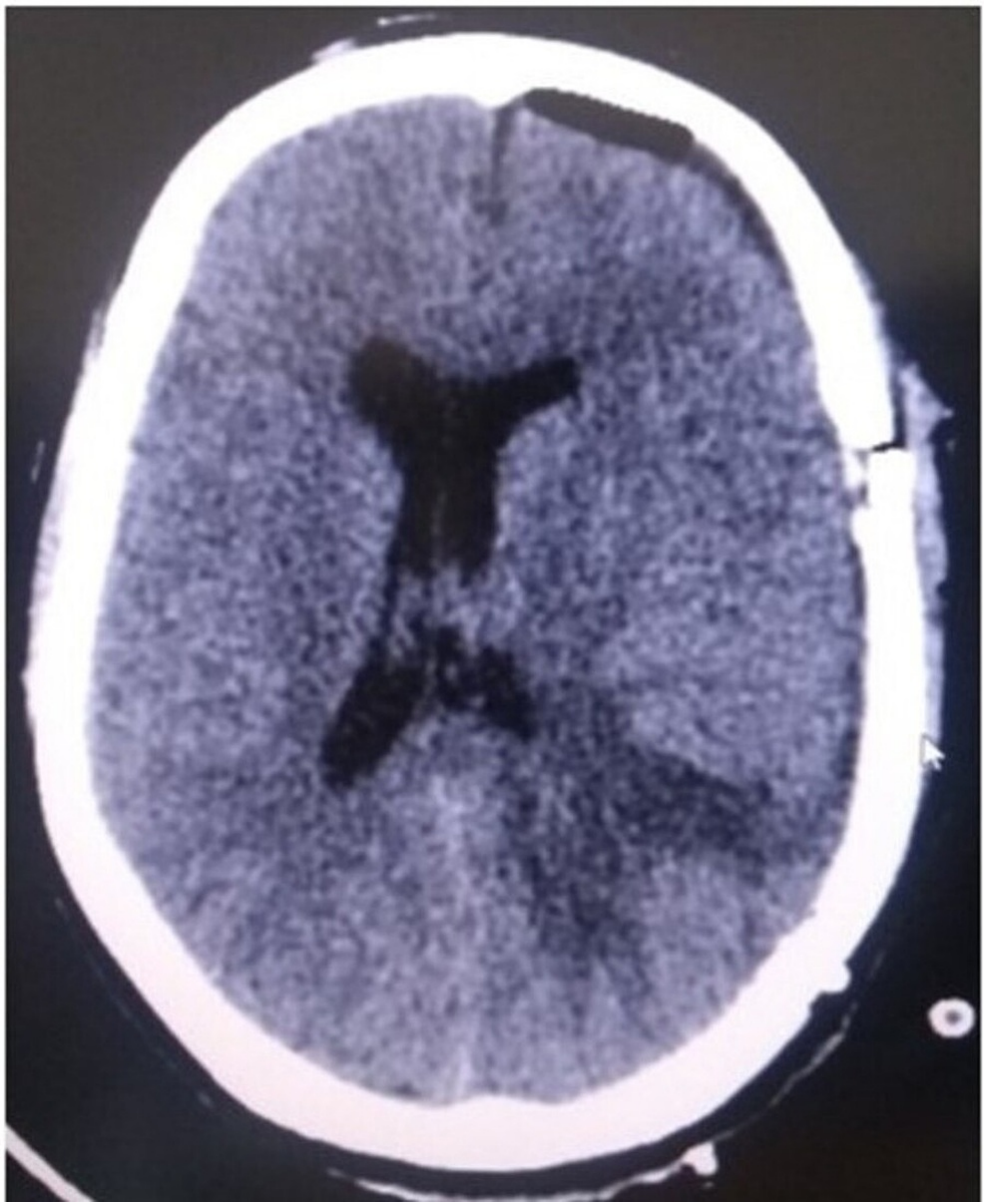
Postoperative CT scan brain - B
